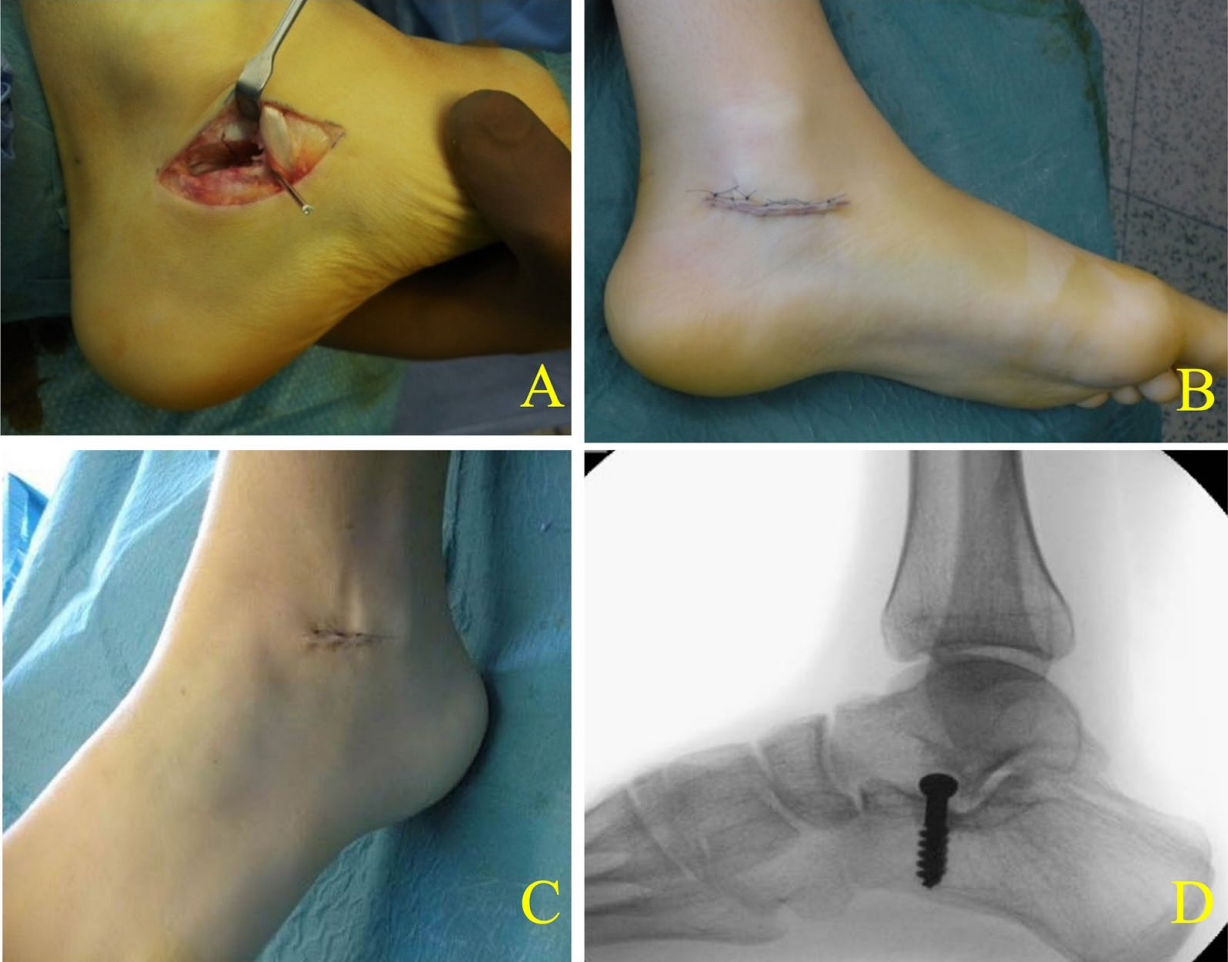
Minimally invasive procedure for TCC resection and hindfoot valgus deformity correction with SESA. A Resection side. B Medial approach incision after skin suture. C Lateral approach incision and skin suture after SESA. D Lateral radiographic view at FU before screw removal
